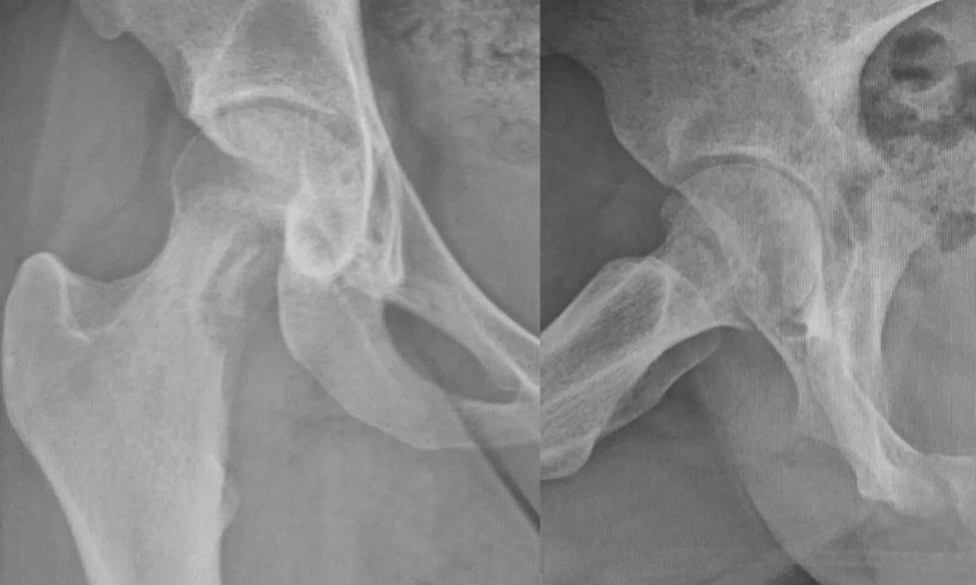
Initial x-ray demonstrating an osteolytic lesion at the femoral neck. AP on the left and lateral on the right side.
